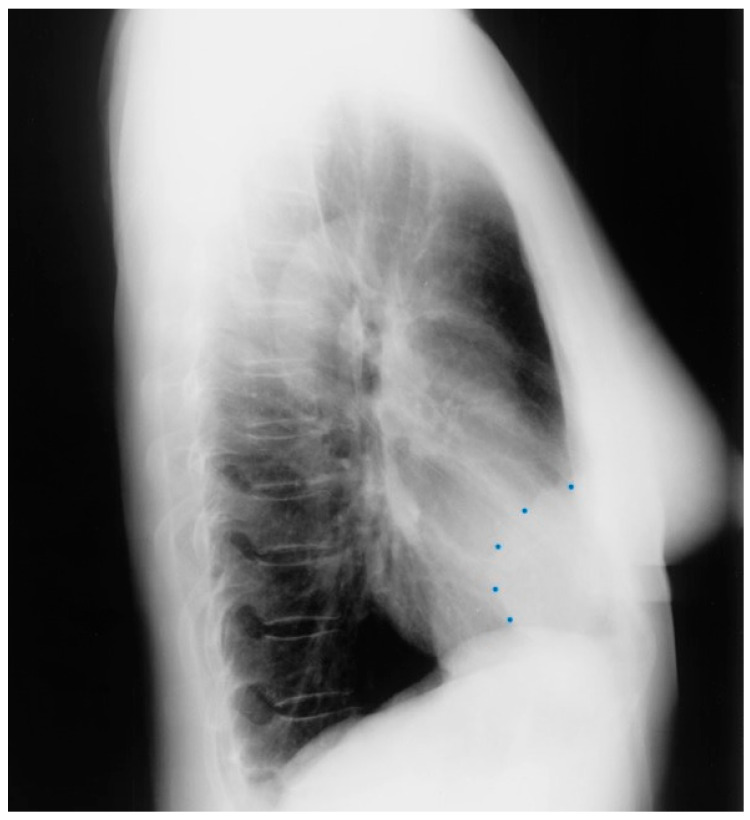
Lateral chest X-ray of a pericardial cyst: This is the lateral chest X-ray of the same-40 year-old female with a right cardiophrenic angle pericardial cyst. This image demonstrates that this pericardial cyst (blue dots) is located anteriorly in the chest and adjacent to the heart, and explains the silhouette sign on the posteroanterior chest X-ray. In this image, because the cyst and the heart are superimposed, their radiodensities are summed and the cyst appears denser than the rest of the heart that it does not overlie.
